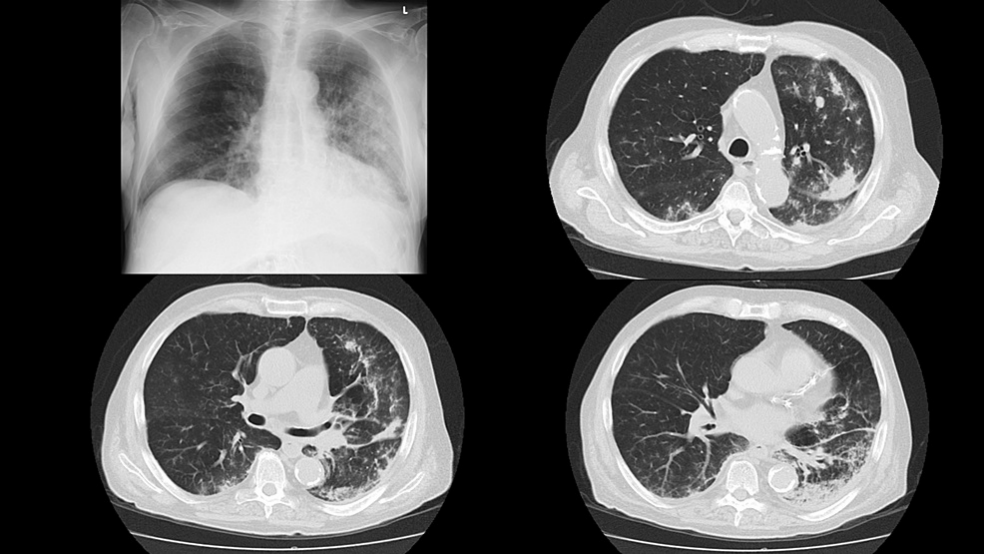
Chest X-ray and chest CT scan on admission Left top: chest X-ray reveals infiltration on the left lung. Right top: upper level of chest CT scan. Left bottom: middle level of chest CT scan. Right bottom: lower level of chest CT scan These CT scans reveal infiltrative shadows on both lungs, mainly on the left and dorsal sites.
